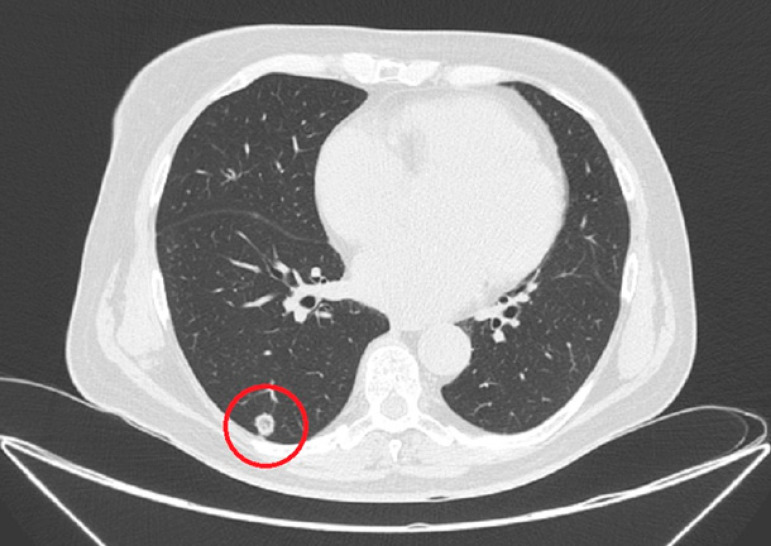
Computed tomography scan of the mass.
